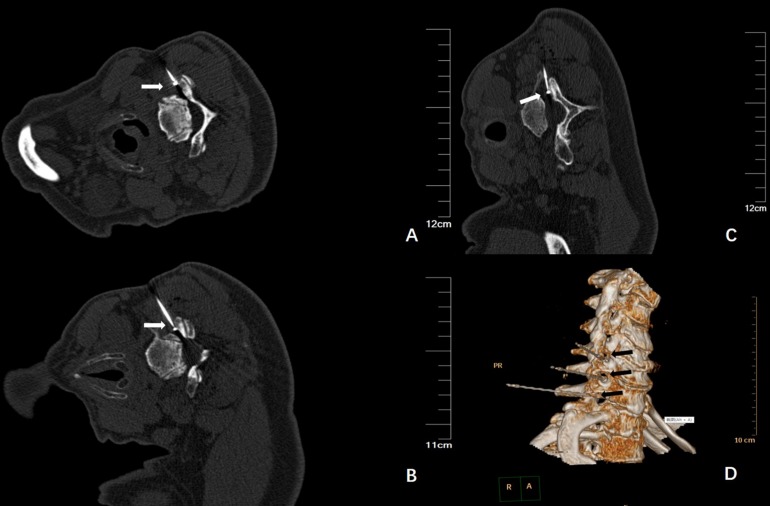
(A) Plain CT scan shows the needle tip was located at the right side of C5-6, as indicated by the arrow; (B) Plain CT scan shows the needle tip was located at the right side of C6-7, as indicated by the arrow; (C) Plain CT scan shows the needle tip was located at the right side of C7-T1, as indicated by the arrow; (D) The three-dimensional reconstruction shows the needle and the direction, located at the right side of the cervical nerve root, as indicated by the arrow.
